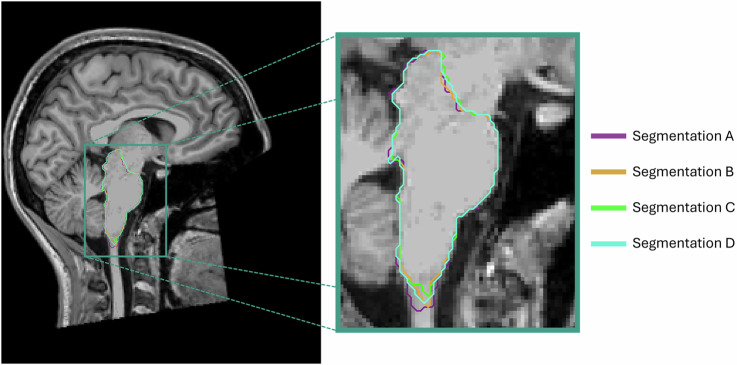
Example of segmentation variability. Different segmentations of the brainstem over a T1-weighted magnetic resonance image. Four different segmentation methods were used for generating these masks, creating slightly different versions of the output
